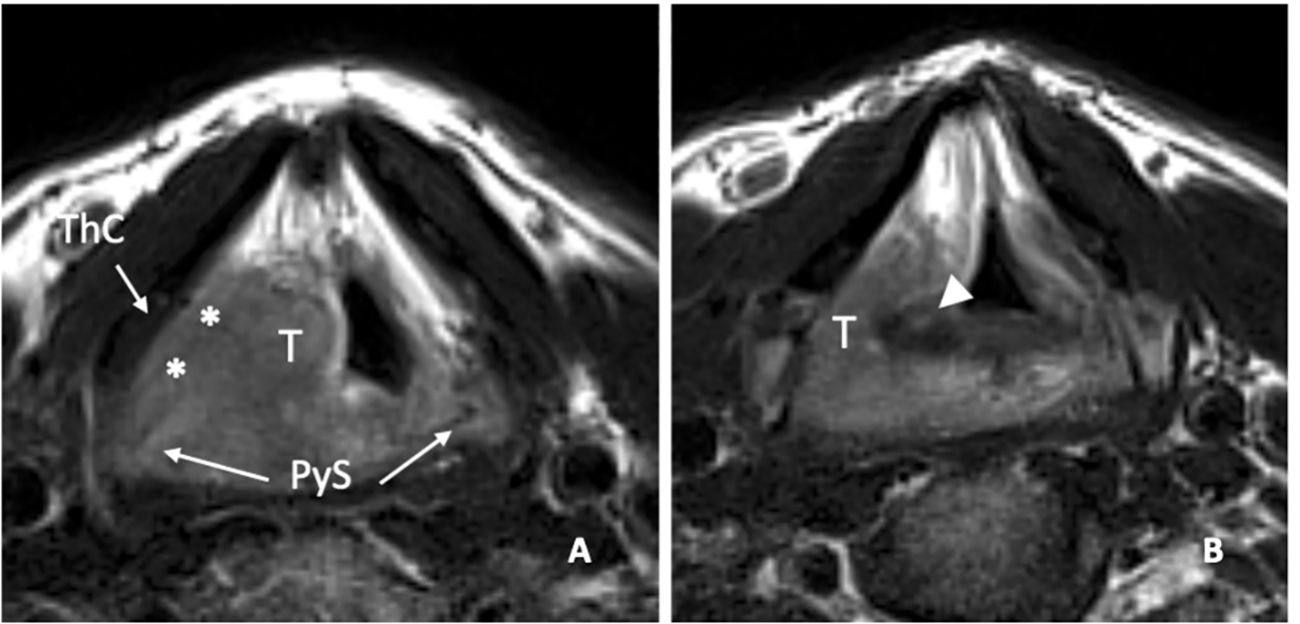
MRI T2-weighted image on axial plane shows a right supraglottic tumour (T) that massively infiltrates the superior paraglottic space (A, asterisks) and involves the right piriform sinus (PyS). The non-ossified thyroid cartilage (ThC) is not involved. The lesion appears indissociable from the right arytenoid (white arrowhead) that shows signal alteration with a sclerotic aspect (B).
